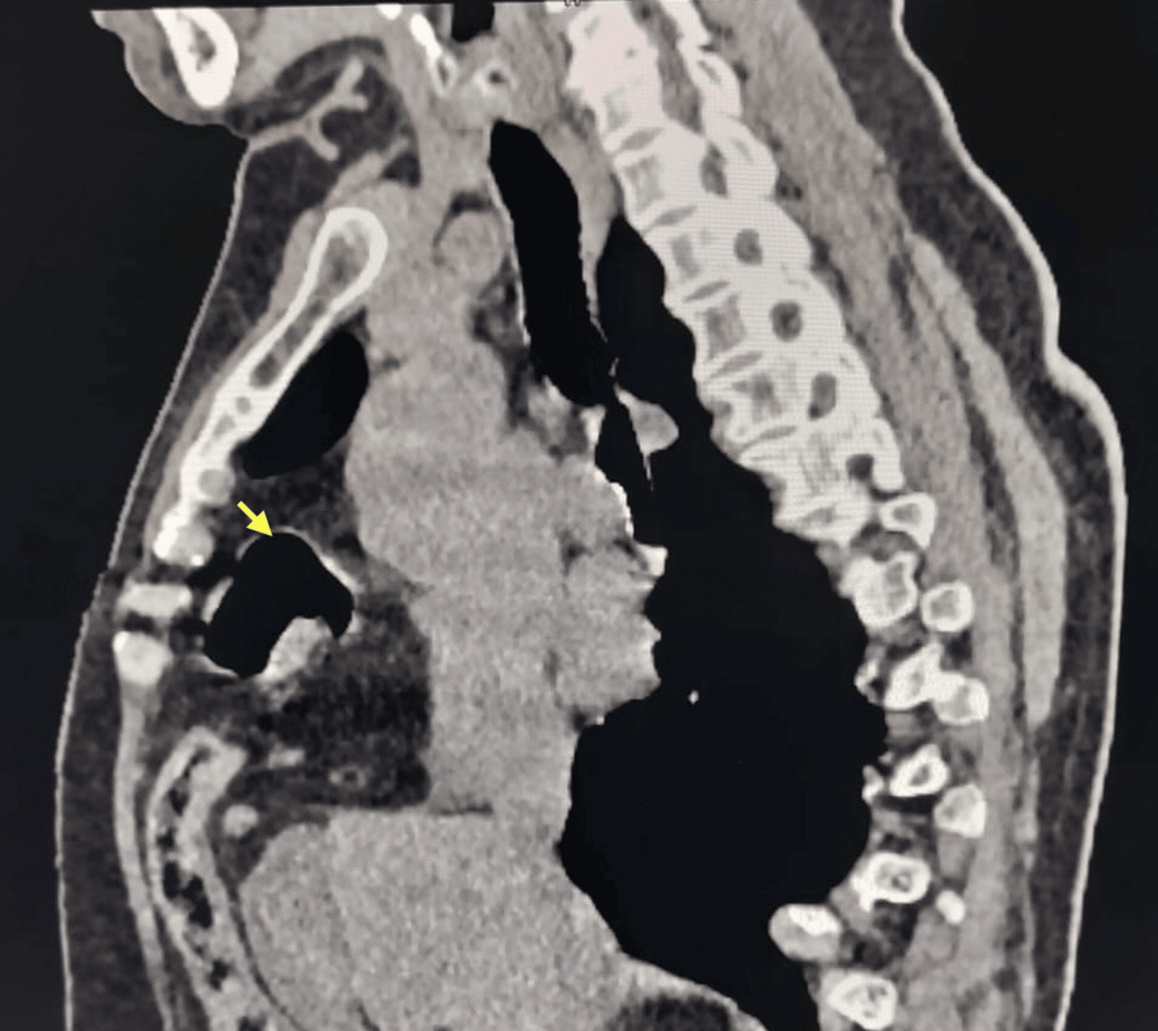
Sagittal view of CT showing the herniation of abdominal content (large bowel, marked with a yellow arrow) into the thoracic cavity.
